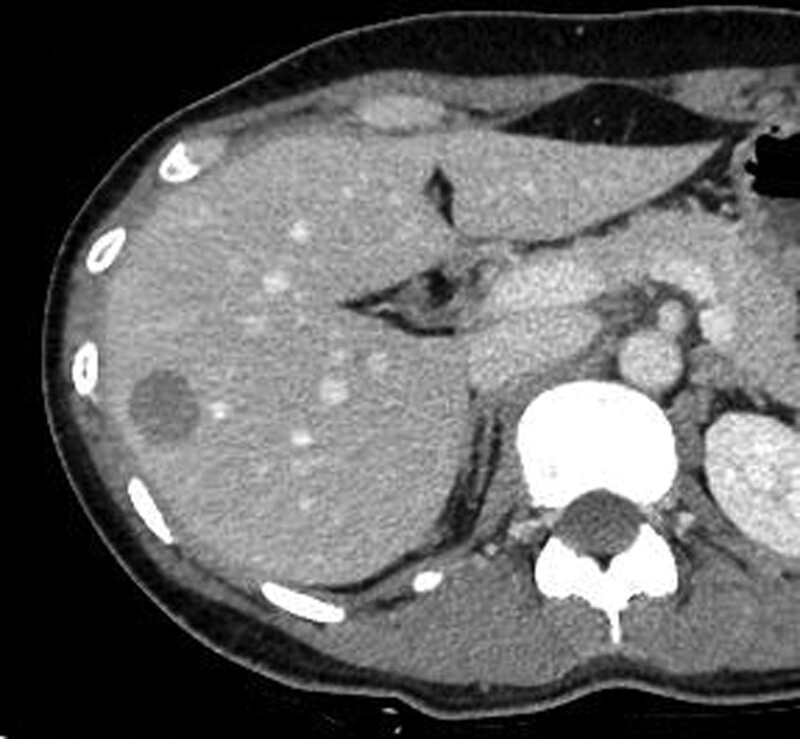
Axial enhanced computed tomography (CT) suggested a 20-mm contrast-impaired area in S5/6 of the liver (yellow arrow). Liver biopsy revealed that this lesion was consistent with angiosarcoma metastasis.
